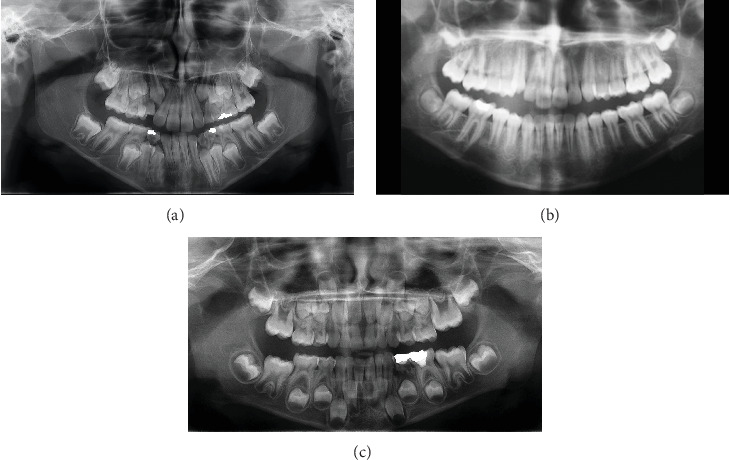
(A) Panoramic radiograph showing dental age of 9.5 years. (B) Panoramic radiograph showing dental age of 10.5–11 years. (C) The panoramic radiograph displays all teeth with open apices.
